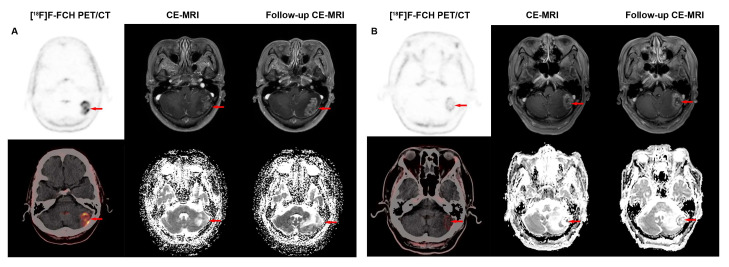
(A) A 64-year-old female patient with BM of lung cancer after SRS underwent follow-up examination without any symptoms. [^18^F]F-FCH PET/CT showed a lesion measuring 2.1 × 1.6 cm with high [^18^F]F-FCH uptake (SUV_max_ = 5.3) in the left cerebellar region. CE-MRI revealed that the lesion showed progressive enhancement and had a low ADC value. After 4 months of follow-up, CE-MRI revealed that the lesion showed significant enlargement and had a low ADC value. The lesion was diagnosed as recurrent neoplasms (red arrow). (B) A 64-year-old male patient developed headache after SRS treatment. An [^18^F]F-FCH PET/CT and MR examination revealed a left cerebellar mass. The left cerebellar mass measured 2.6 × 1.9 cm with an SUV_max_ value of 1.43. CE-MRI revealed that the lesion showed progressive enhancement and had a low ADC value. After 8 months of follow-up, CE-MRI revealed that the lesion showed no significant size changes and had a low ADC value. This lesion was finally confirmed to be a non-recurrent lesion (indicated by a red arrow).
